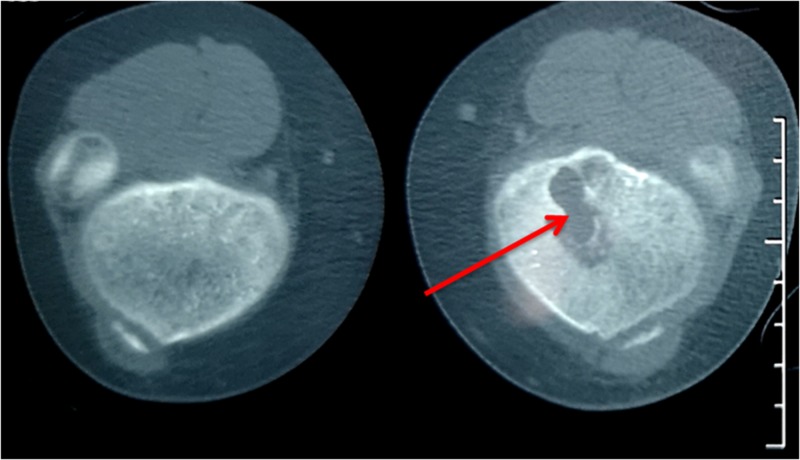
Knee CT: lytic lesion in the proximal left tibia (red arrow)
